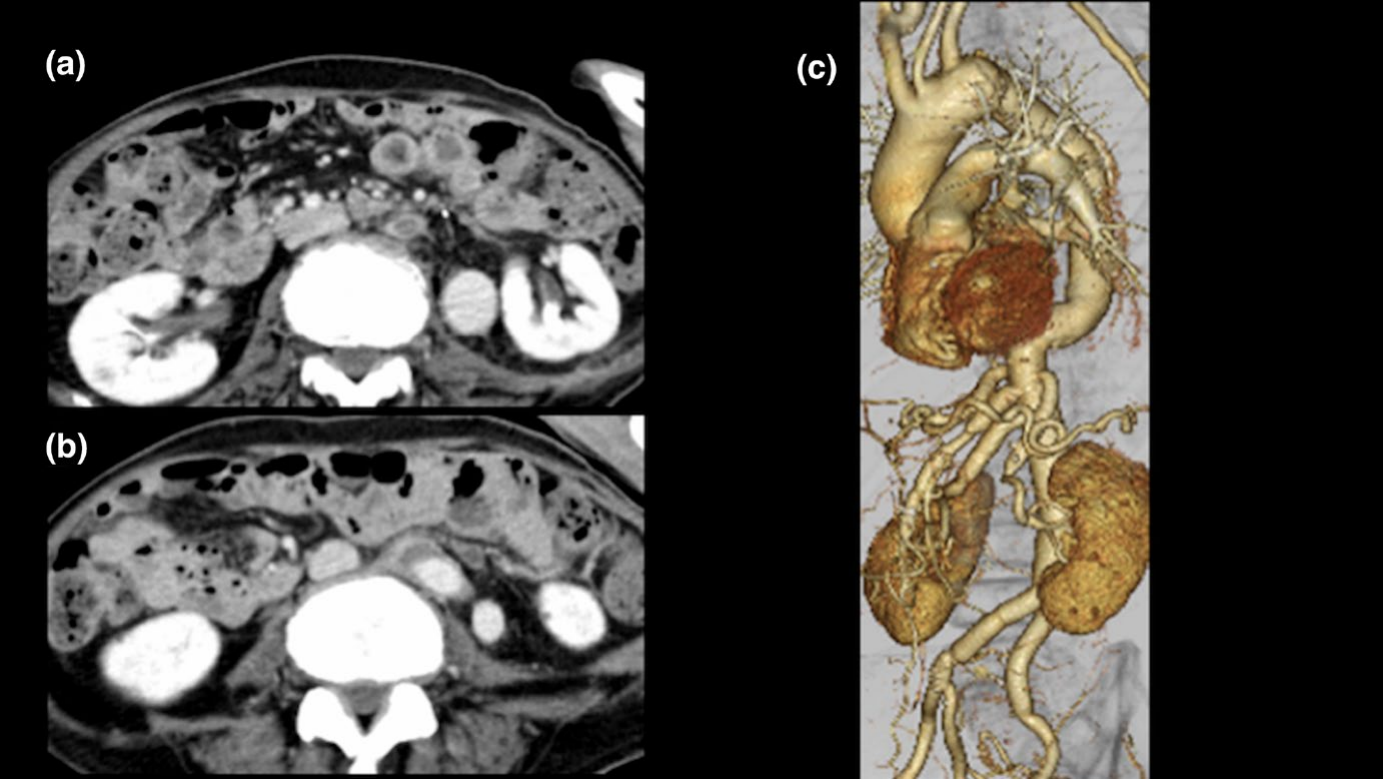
Postoperative computed tomography scan obtained at the time of discharge. (A, B) The scan shows the retroperitoneal space without fluid retention and (C) without any anastomotic complications
